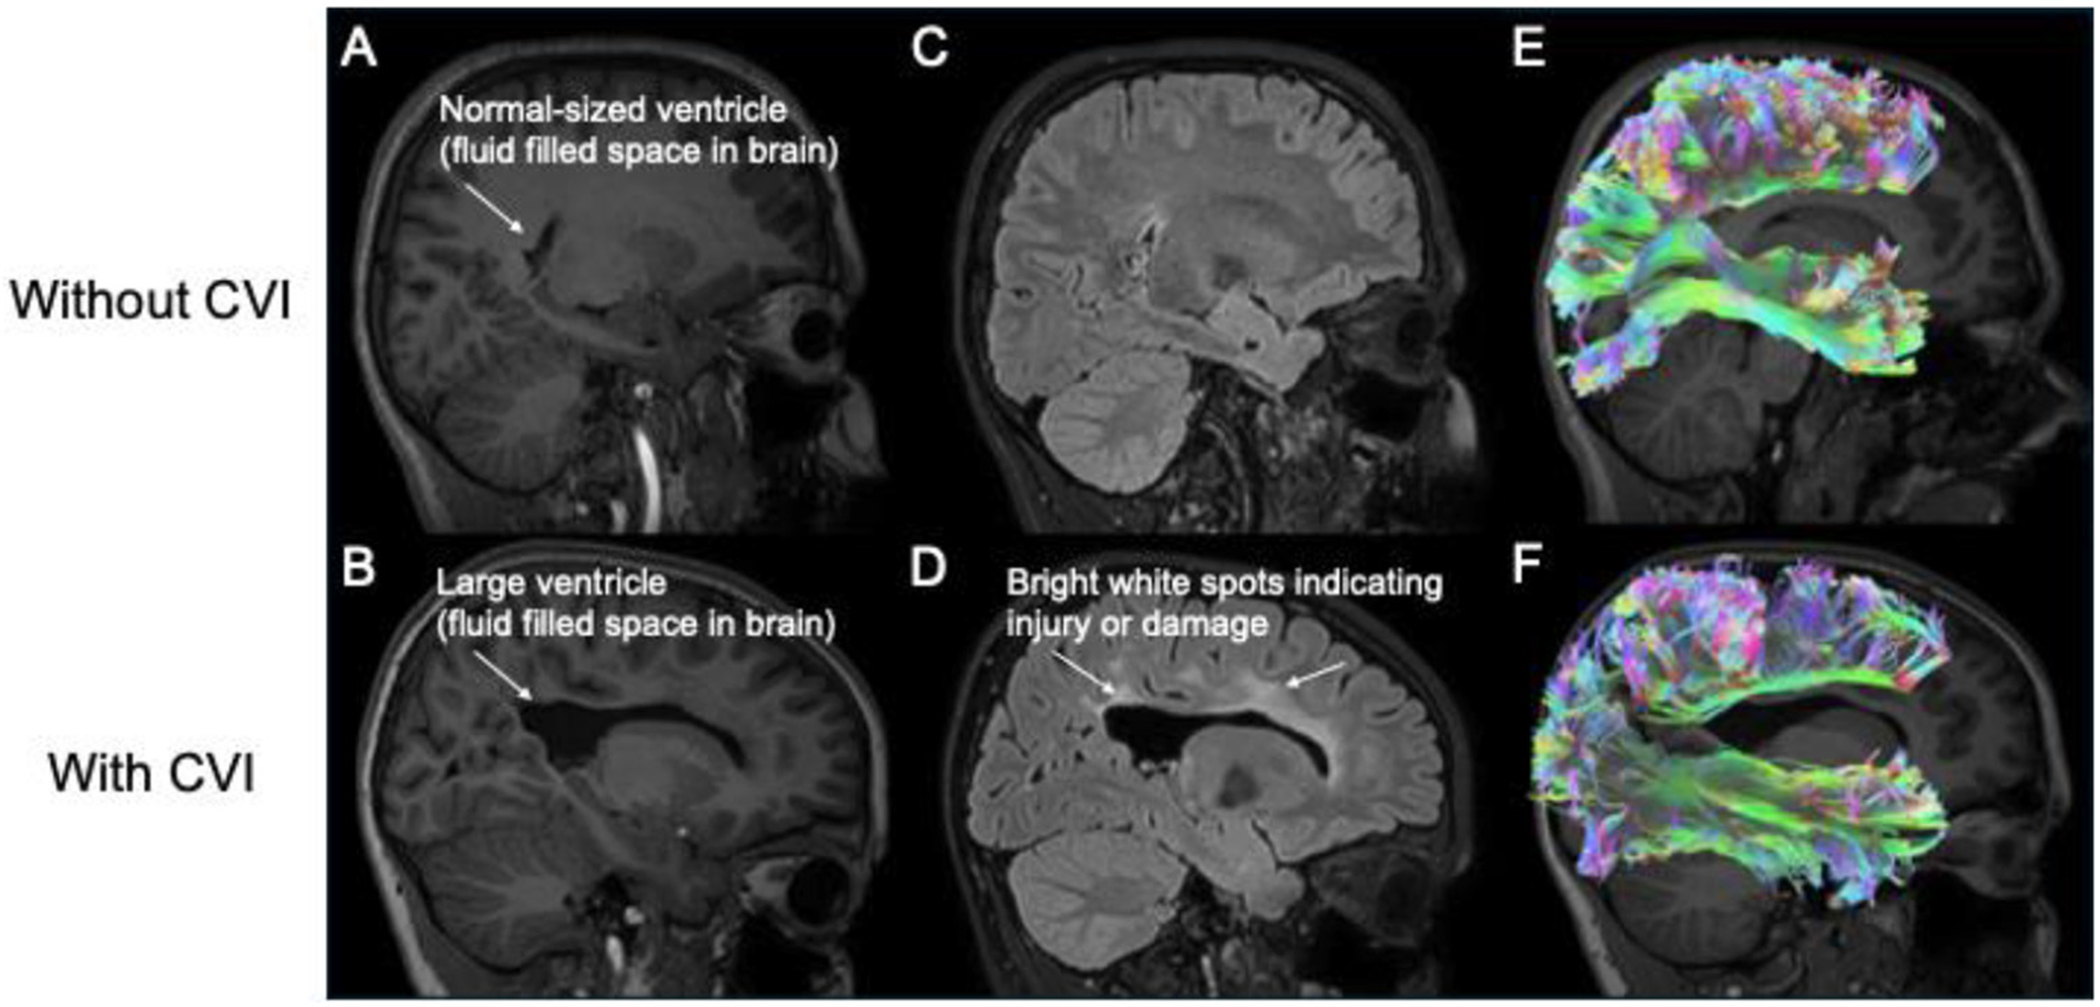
How we use MRI to look at the brain structure, areas of injury, and pathways. The eyes and nose on the right side of each image. (A, C, E) A 12-year-old without CVI. (B, D, F) A 10-year-old with CVI who was born early and had brain bleeds around the time of birth. (A, B) Large black space in the middle of the brain (arrow). (C, D) Bright white spots suggest areas of injury or damage [arrows in (D)]. (E, F) Examples of brain pathways used to process visual information. In CVI, these pathways can be damaged or smaller.
